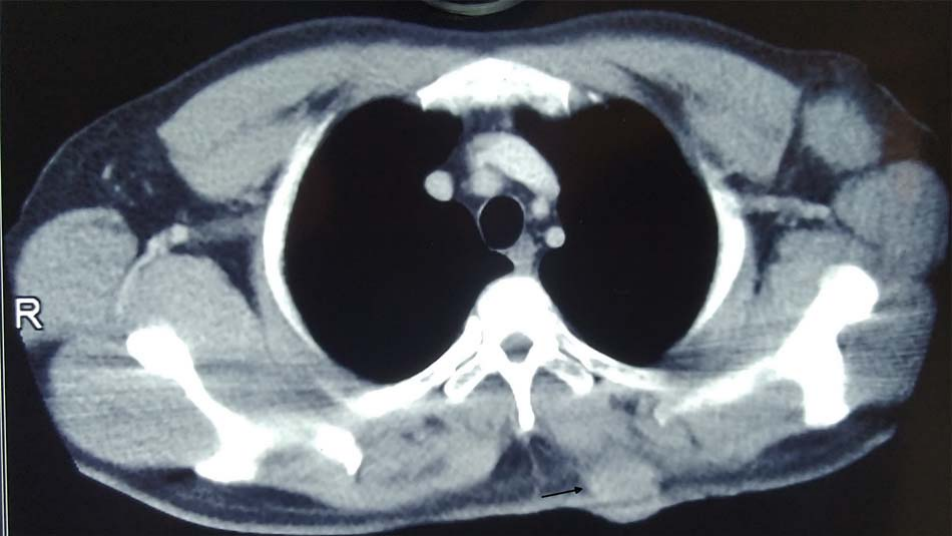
CT image showing well defined lesion in skin and subcutaneous tissue (arrow).
